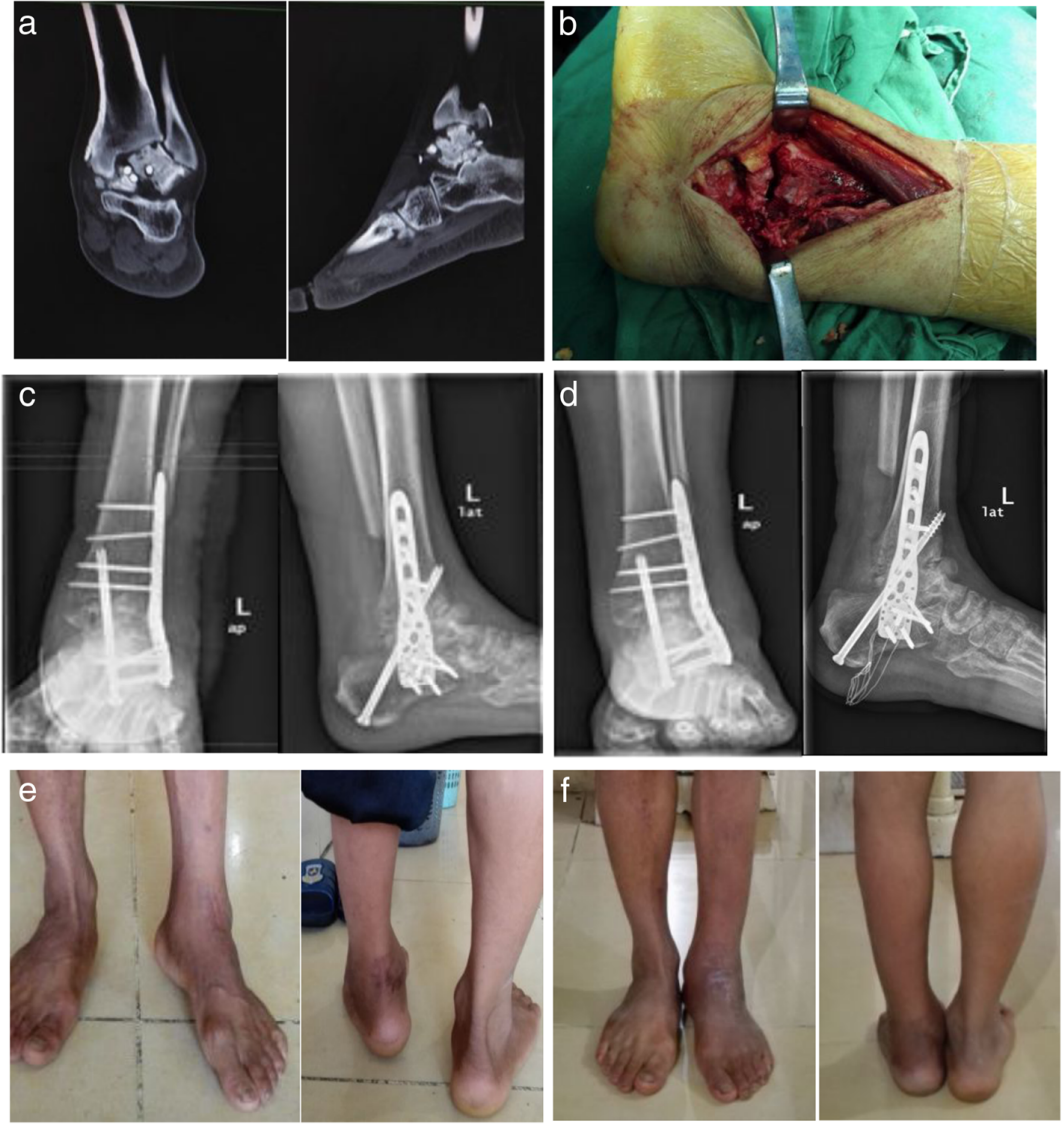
The male case, 57 years old, with left talus necrosis after internal fixation of fracture and dislocation, was treated with TTCA. a Preoperative CT showed avasculanecrosis of talus dislocation with old fracture and internal fixation. b Through the lateral incision, tibiotalar and subtalar joint were exposed after fibular was cut 7-8 cm above the distal fibula. c Postoperative X-ray. d X-ray 12 months after operation. e Left ankle shows obvious inversion deformity before operation. f Left ankle shows nearly normal 12 months after operation
